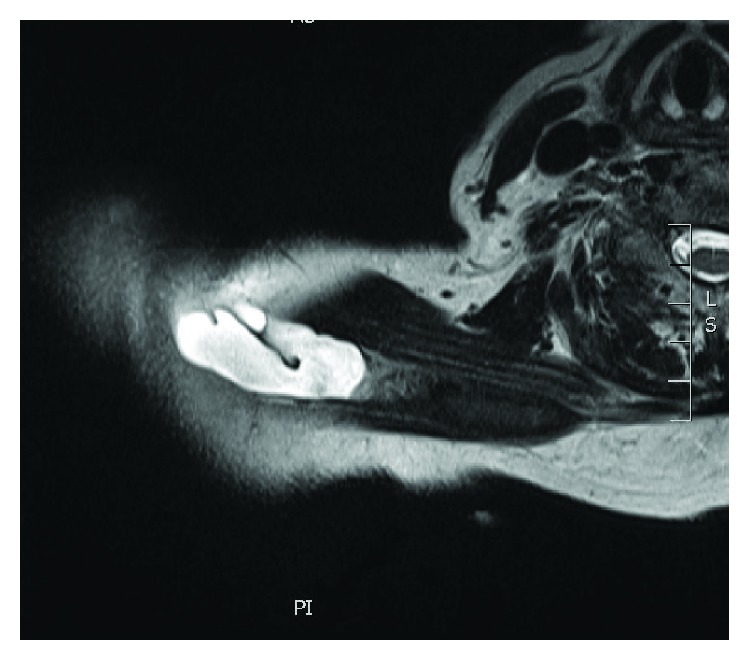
Axial cuts of MRI of the right thorax depicting cyst measuring 2 cm (AP) × 13 (transverse) × 1.8 cm (craniocaudad).
